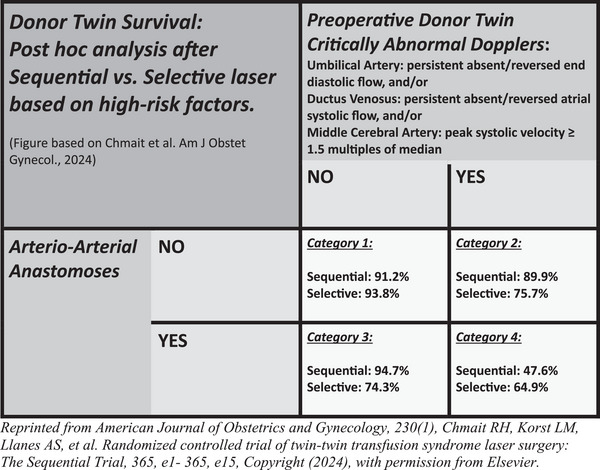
Donor twin survival by laser technique and risk category. The proportion of the Sequential Trial patients with donor twin survival in each of four categories (based on critically abnormal Doppler [CAD] parameters and the presence of arterioarterial anastomoses [AA]) by sequential versus selective surgical techniques. The categories are as follows: Category 1, no CAD and no AA; Category 2, CAD with no AA; Category 3, no CAD with AA; and Category 4, CAD with AA.
